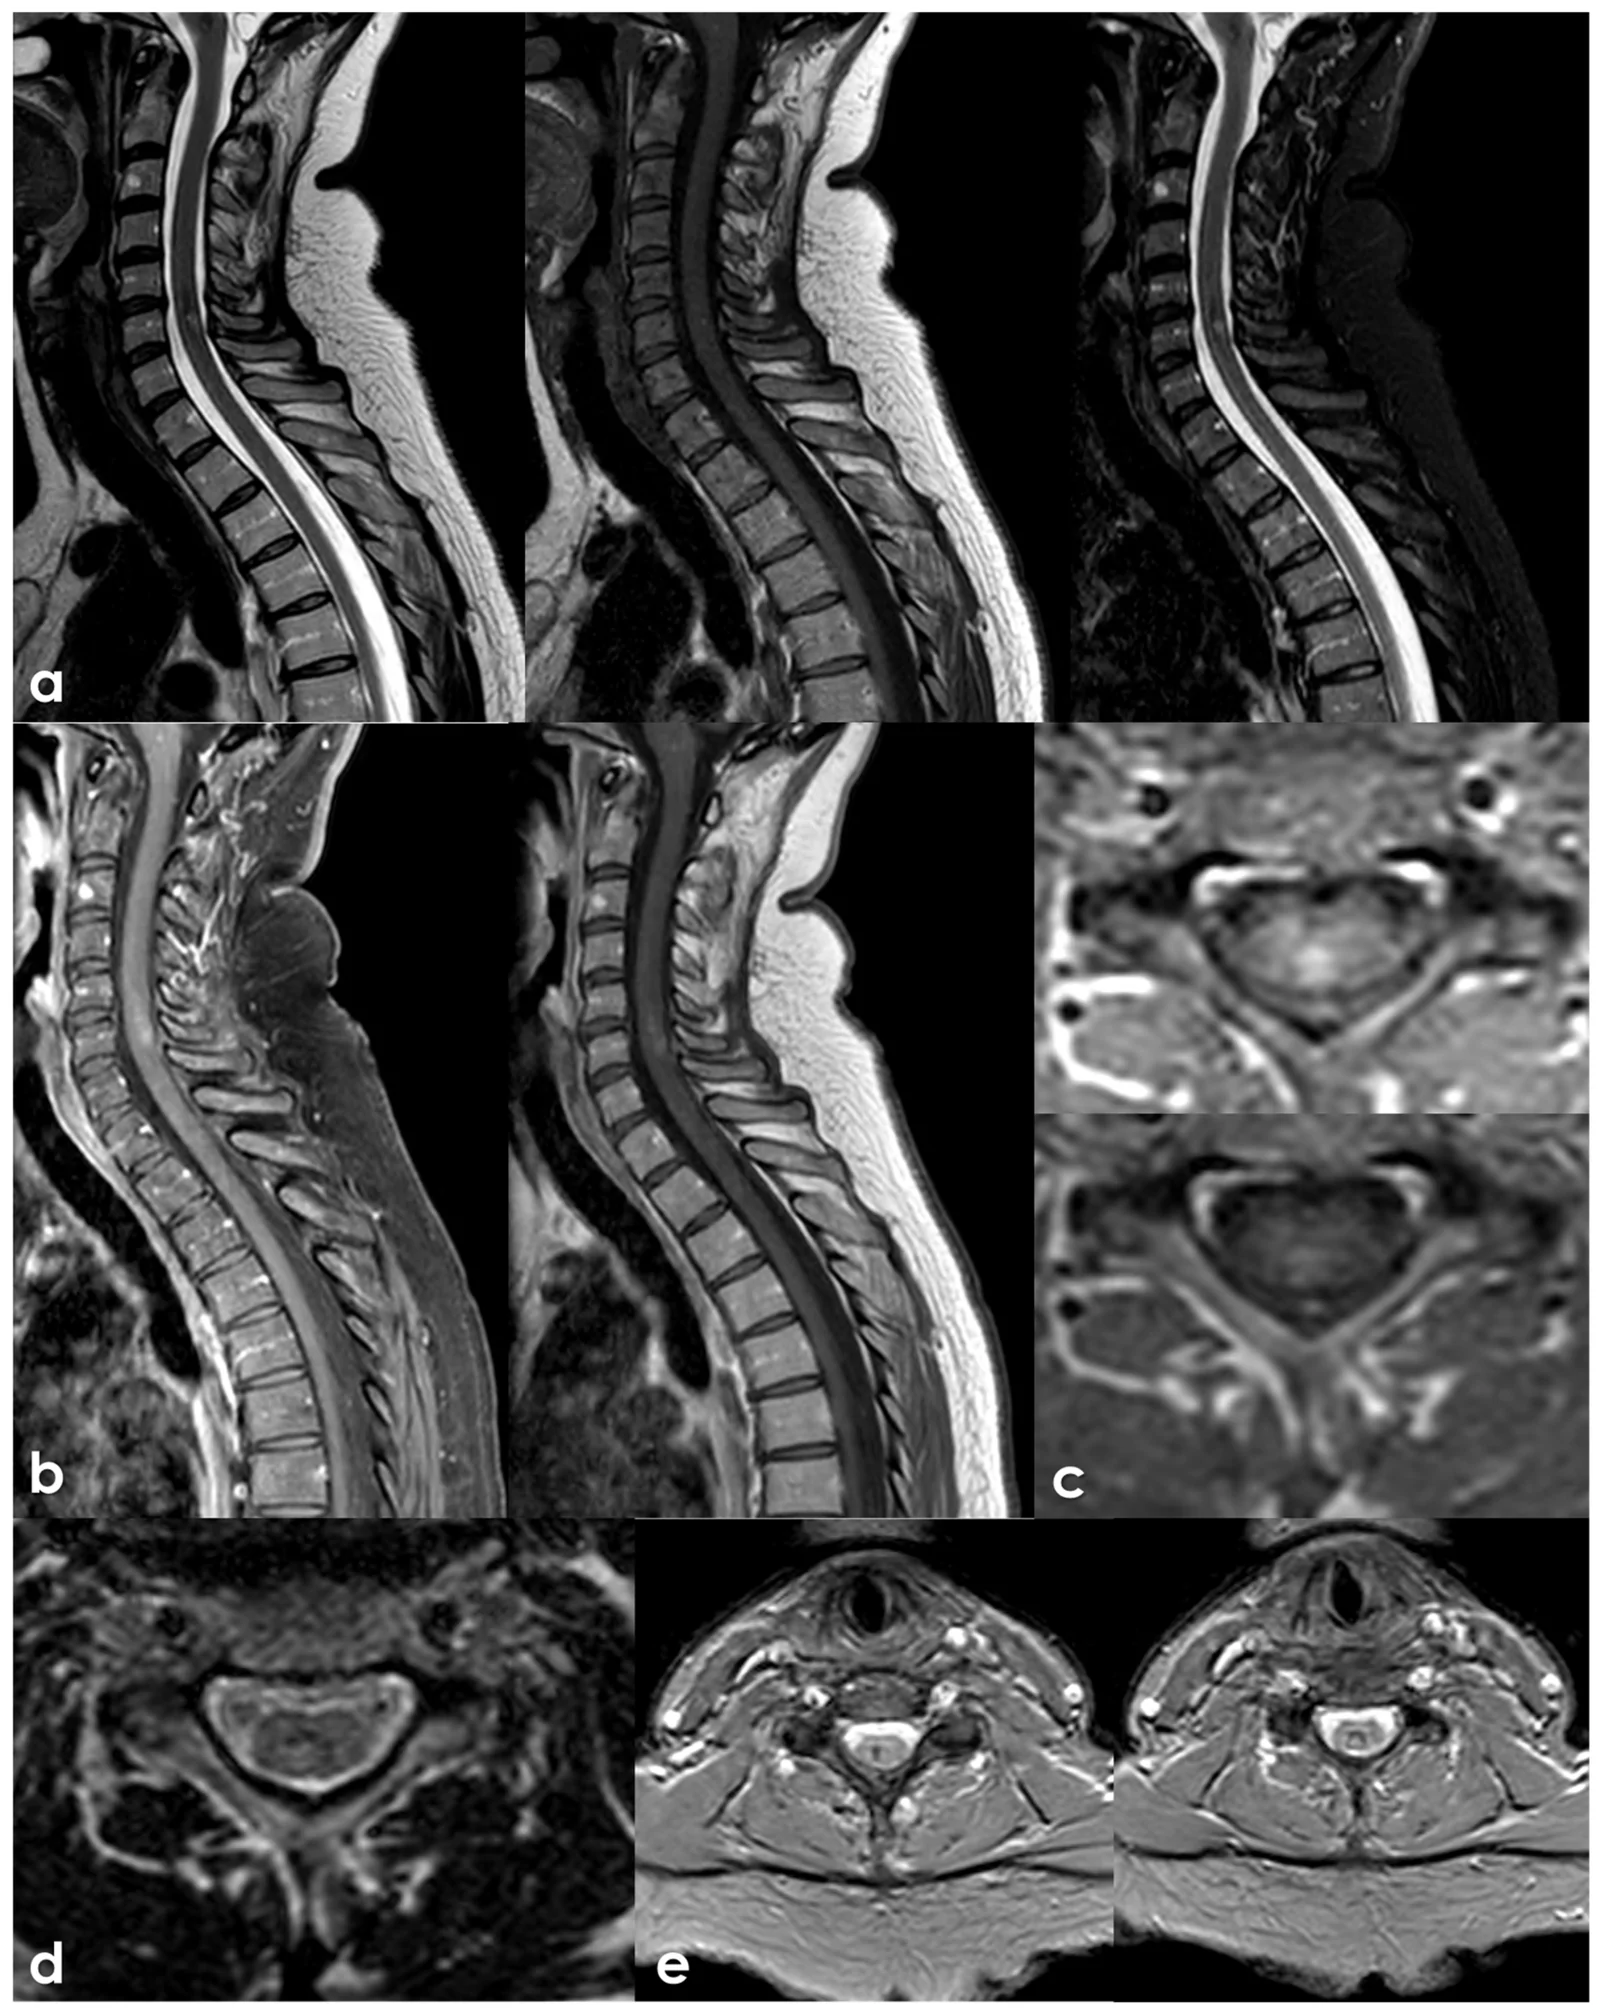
Spinal cord MRI in the same patient of Figure 5, performed about 45 days after the onset of ataxic gait and neurological pattern of posterior funiculus involvement with a cervical level. (Panel a): Sagittal T1W, T2W and STIR sequence (from (left) to (right)), showing a mildly T1-hyperintense lesion in the posterior half of the cervical spinal cord. (Panel b): Same lesion in sagittal post-gadolinium T1 Dixon sequence, showing intralesional contrast enhancement with a homogeneous pattern and smooth lining. (Panel c): Same appearance of panel b in axial plane, confirming the posterior median-paramedian location of the lesion. (Panel d): Axial T2W image showing a slightly hyperintense lesion with a hypointense border in the posterior funiculus. (Panel e): Axial GRE sequence with a corresponding hypointense signal. The findings are consistent with a cervical intramedullary SCCM with late subacute bleeding. The patient had a known diagnosis of FCCM due to a frameshift mutation of CCM2 gene, but spinal cord was not screened as potential site for cavernous malformation at the time of diagnosis. About 20 years after the diagnosis of FCCM, the patient developed a subacute myelopathy, leading to the identification if spinal bleeding due to an underlying cavernous angioma.
